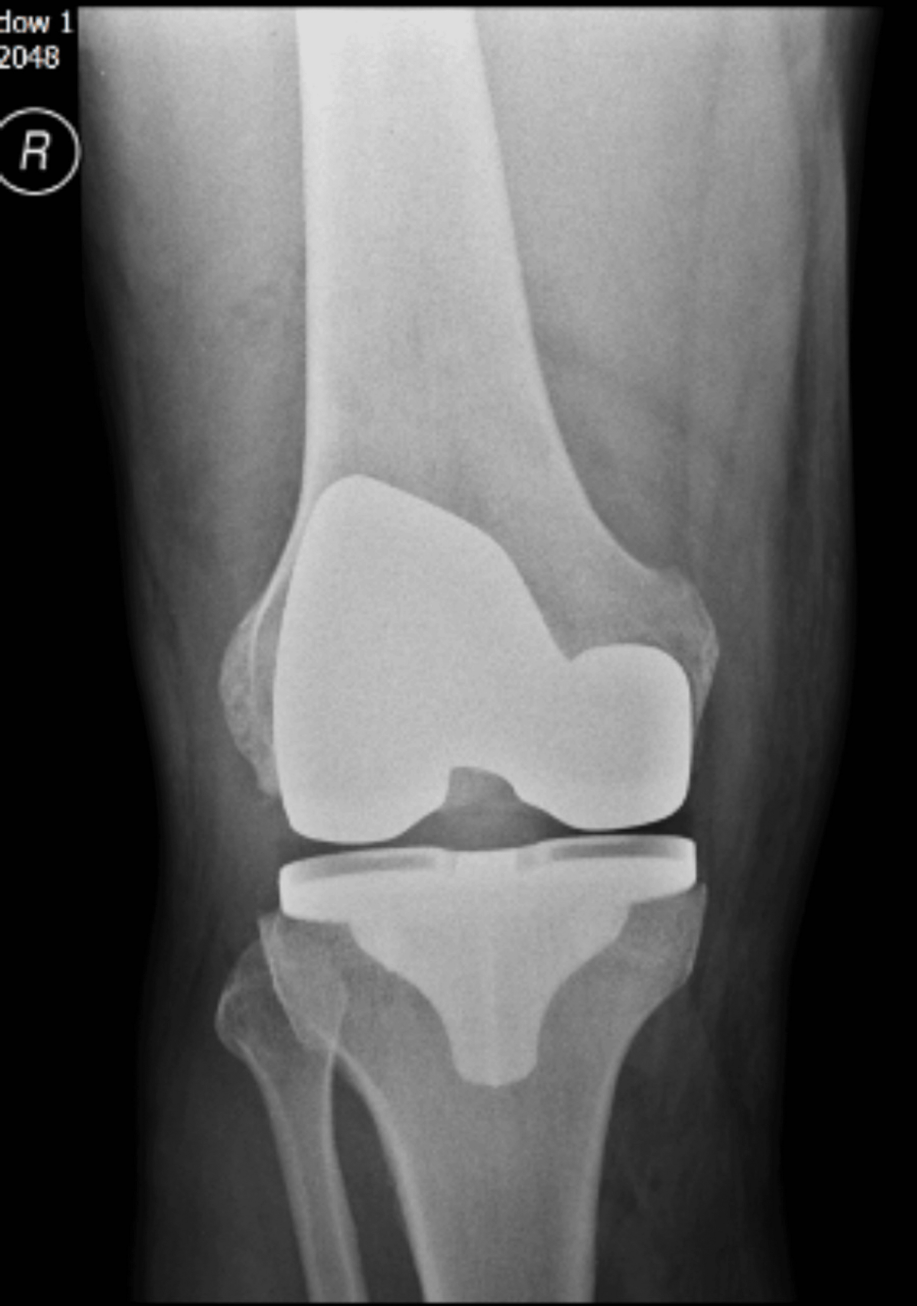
Postoperative anteroposterior radiograph of the knee showing total knee replacement in place and gross joint effusion in the form of loss of the supra and infrapatellar fat pad.
